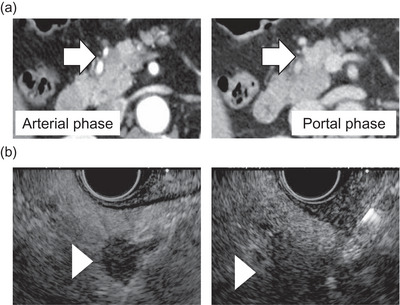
(a) Arrows (⇒) indicate primary pancreatic cancer in a CT scan. (b) Preoperative EUS‐FNB for pancreatic tumor. Arrowheads (▷) indicate primary pancreatic cancer. CT, computed tomography; EUS‐FNB, Endoscopic ultrasound‐guided fine‐needle biopsy.
